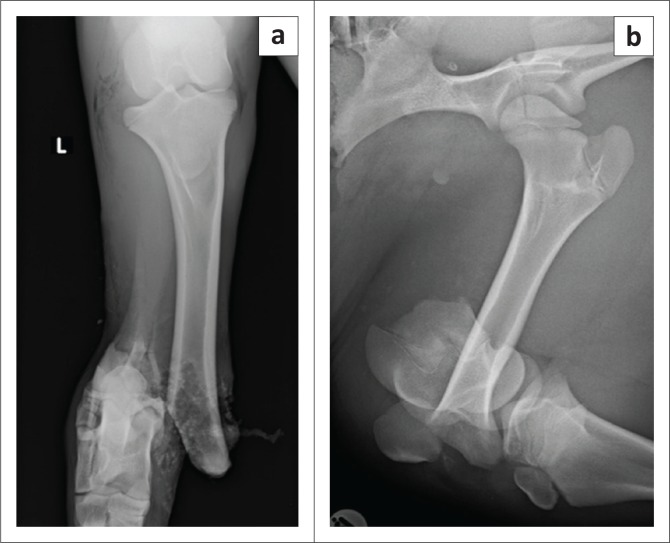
Radiographs of the pelvic limbs of a 4-month-old wildebeest (*Connochaetes taurinus*) showing complete fractures to the (a) left tibia and (b) right femur sustained in a motor vehicle accident.
